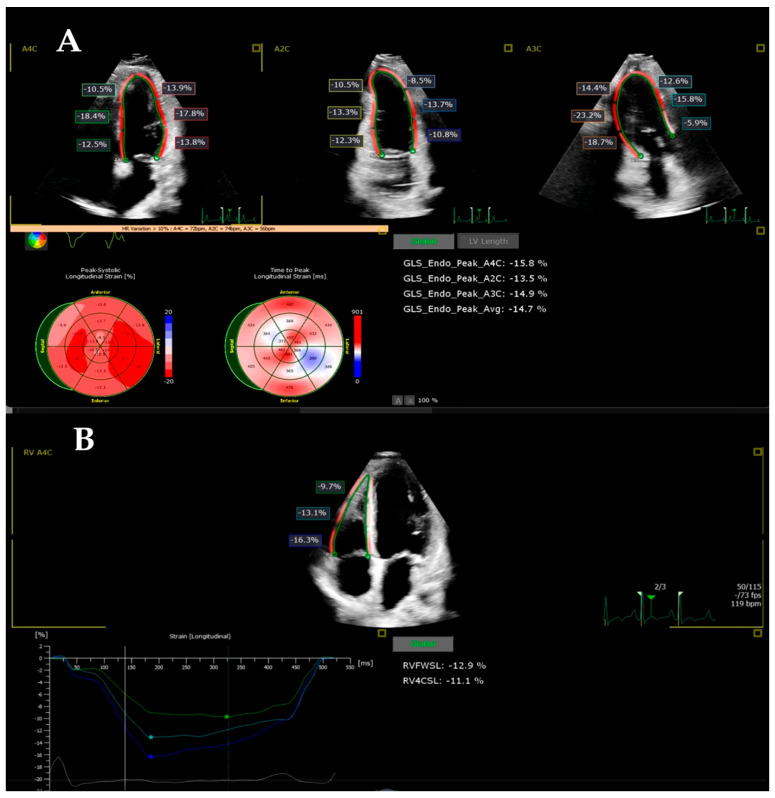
Quantification of left and right ventricular longitudinal strain image. (A) Left ventricular global longitudinal strain (LVGLS) analysis from apical 4-, 2-, and 3-chamber views. Strain values are diffusely reduced (average LVGLS-14.7%), indicating impaired systolic function. The bull’s-eye plot highlights segmental reduction, predominantly in the infero-septal and anterior segments. (B) Right ventricular free-wall longitudinal strain (RVFWLS) and 4-chamber strain analysis demonstrating decreased RV systolic function (RVFWLS-12.9%, RV4CSL-11.1%).
